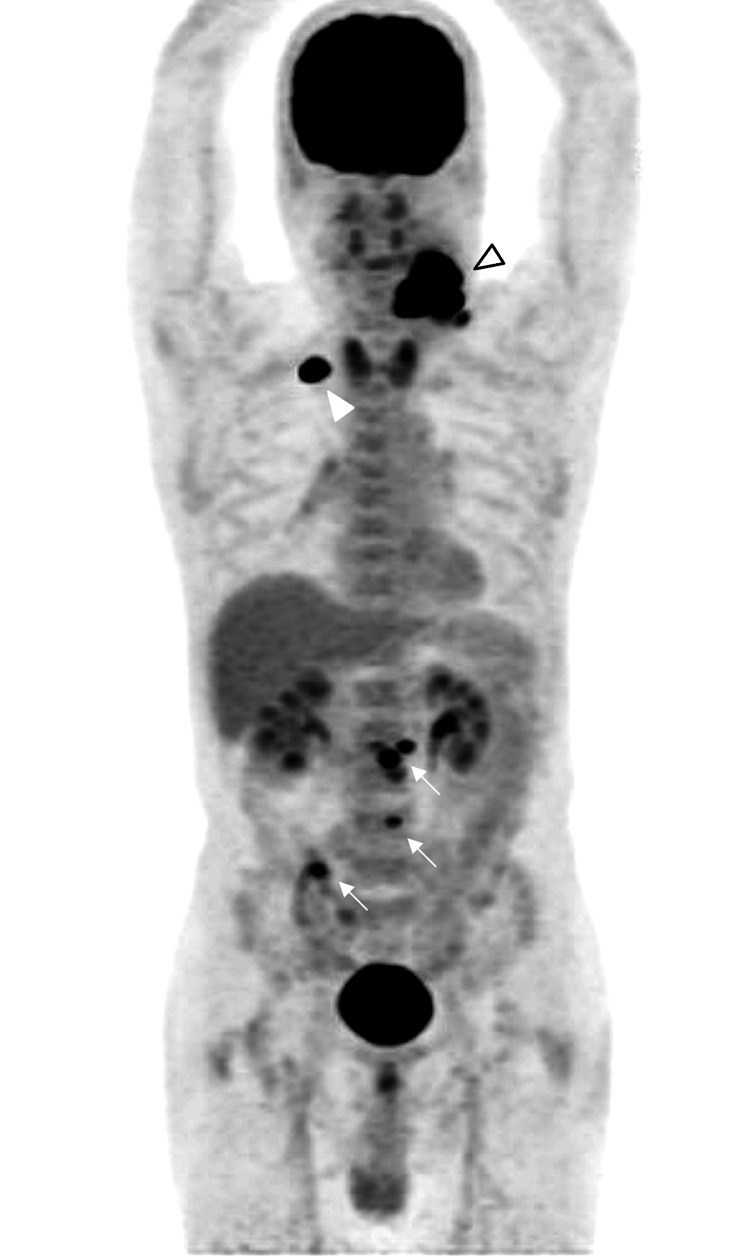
Positron emission tomography (PET) with FDG integration A coronal view of a positron emission tomography (PET) scan with FDG integration shows a right lung metastasis (white triangle), cervical lymph node metastases (black framed white triangle), and multiple lymph node metastases (arrows).
